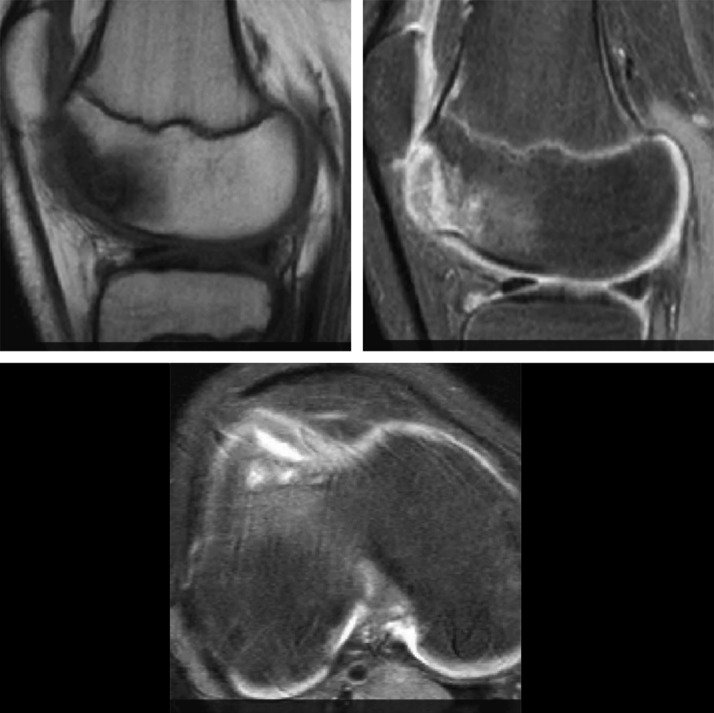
Knee MRI image. The low signal in the T1-weighted image and the high signal in the T2-weighted image.
